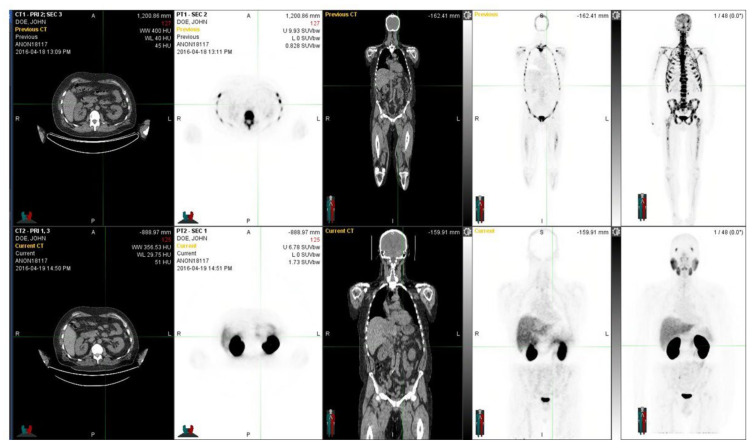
The figure demonstrates a 60-year-old prostate cancer patient diagnosed five years earlier with prostate cancer and T3bN0M0 with an initial PSA 13 and a GS 7. Despite the radical prostatectomy and hormonal therapy, he developed skeletal metastases. He was treated with chemotherapy and multiple targeted bone therapies, abiraterone, and Ra-223 radionuclide therapy. In the upper row, NaF-PET demonstrates a widespread skeletal disease in the whole skeleton, whereas in the lower row, with Ga-PSMA, fewer skeletal metastases are seen. Regions differed significantly from each other, and the PET tracers showed different overall distributions. Fluoride typically targets the cortical bone and bone formation, whereas PSMA targets active cancer cells, preferably in the bone marrow. At the time of imagings, the S-PSA was 0.35 (patient 7).
